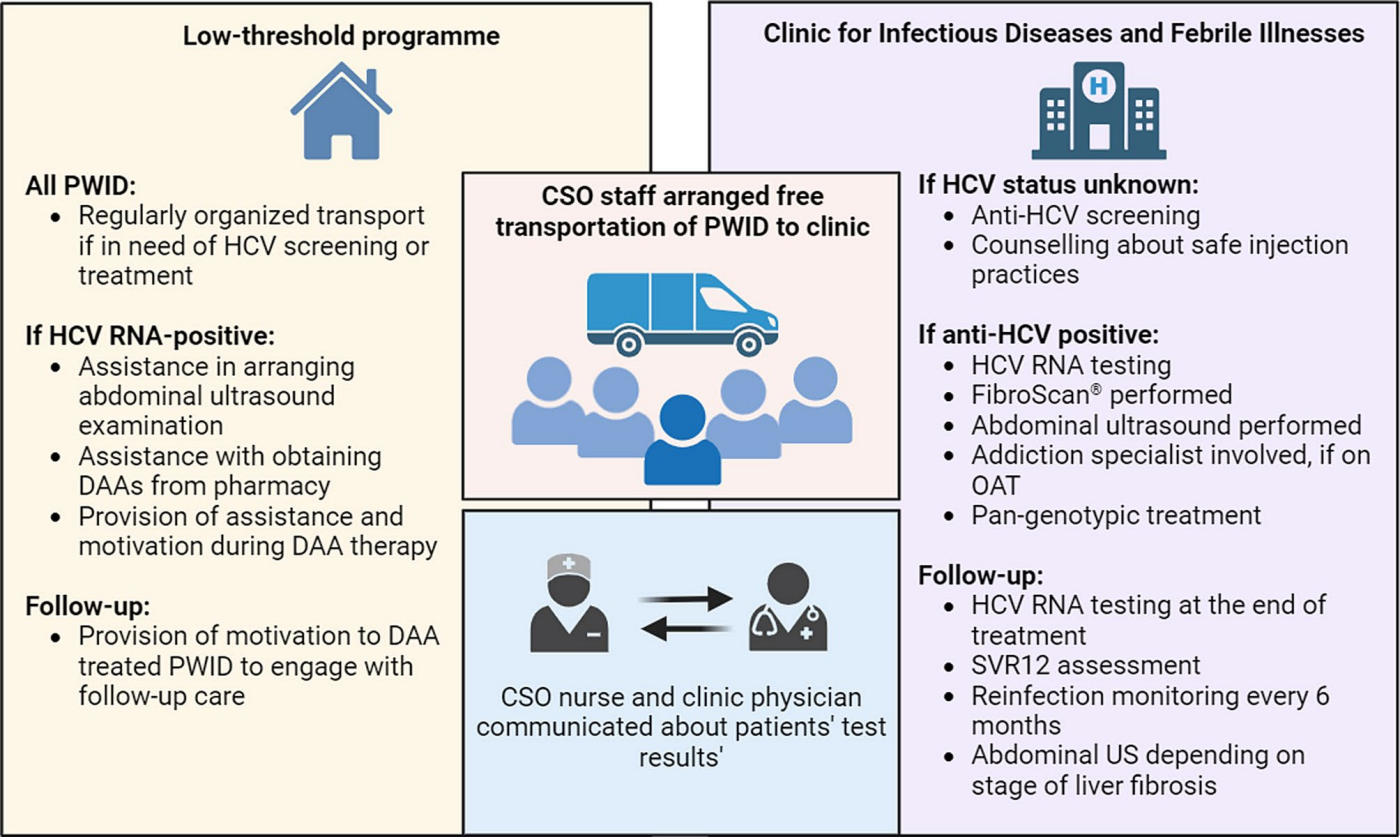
A model-of-care for the management of hepatitis C virus infection in people who inject drugs via the low-threshold programme. Created with BioRender.com. Abbreviations: CSO – civil society organisation, DAA – direct-acting antiviral, HCV – hepatitis C virus, OA T– opioid agonist treatment, PWID – people who inject drugs, SVR12 – sustained virological response 12 weeks after the end of treatment, US – ultrasound
